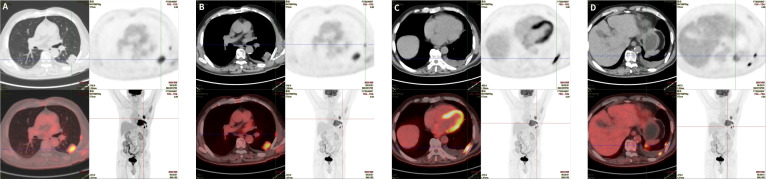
PET/CT findings. (A) Hypermetabolic mass-like lesion in the dorsal segment of the left lower lobe with markedly increased FDG uptake (SUV_max_, 9.07), suggestive of a peripheral lung malignancy with invasion of the adjacent pleura. (B, C) Multiple focal thickenings of the left parietal and visceral pleura showing increased FDG uptake (SUV_max_ range, 3.40–13.28), consistent with pleural metastases. (D) Focal increased FDG uptake in the anterior portion of the left seventh rib, suspicious for bone metastasis.
